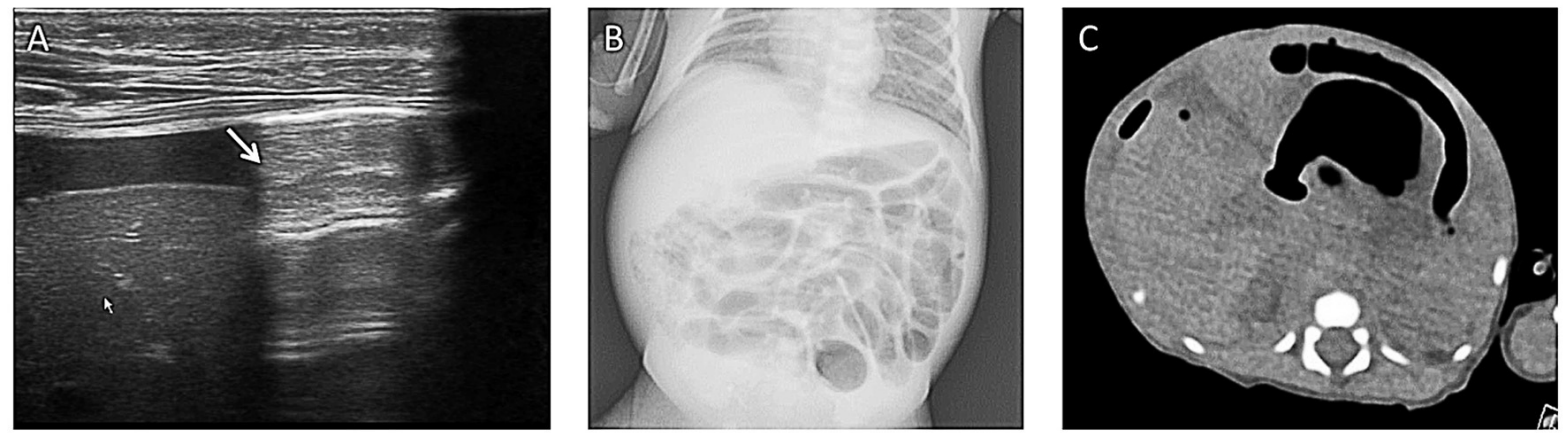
Pneumoperitoneum. A boy (gestational age of 32 weeks) developed abdominal distension and bloody stool on his 14th day. (A) Grayscale ultrasound shows an echogenic interface just below the abdominal wall (arrow) with dirty posterior acoustic shadowing that is from free air in the abdomen. (B) This air was not visible on the corresponding supine abdominal radiograph. (C) The following CT examination supported the result of AUS. This case demonstrates that ultrasound is more sensitive to pneumoperitoneum.
